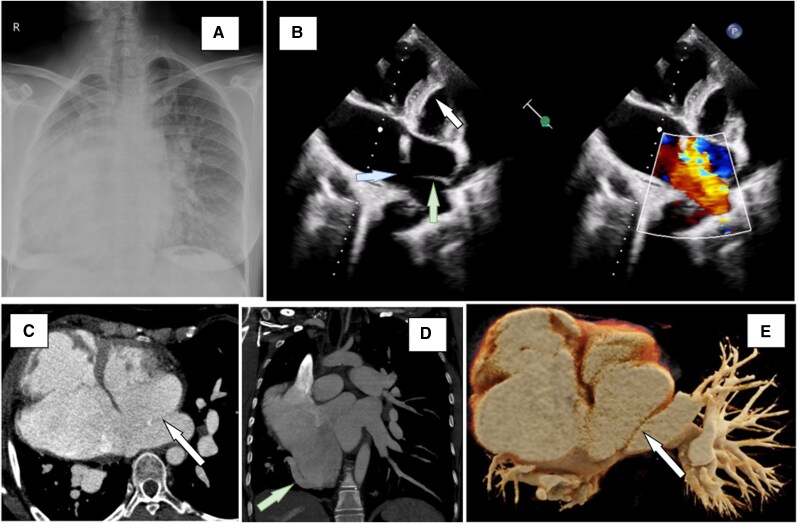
A 38-year-old woman presented with bilateral pedal edema. The chest X-ray postero-anterior view (A) shows cardiomegaly with the cardiac shadow occupying the majority of the right hemithorax. The 4-chamber view of the echocardiogram (B) shows a thin membrane in the left atrial cavity (green arrow) along with a large ostium secundum atrial septal defect (blue arrow). The cardiac computed tomography images (C and D) demonstrate mesocardia, left atrial membrane, a large atrial septal defect, and a dilated right atrial cavity due to left-to-right shunt via the atrial septal defect. Note is also made of anomalous drainage of the right lower lobe pulmonary vein near the right atrium-inferior vena cava junction (green arrow in D). Cinematic virtual reconstruction technique image (E) shows the cor-triatriatum membrane (white arrow).
